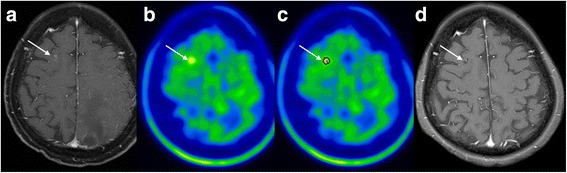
Imaging of a 75-year-old woman who underwent gamma knife radiosurgery (GKR) at a dose of 18 Gy for metastatic brain tumor that originated from lung adenocarcinoma. a T1-weighted MR image with gadolinium enhancement showing contrast-enhanced lesion in the right frontal lobe (*arrow*). b
